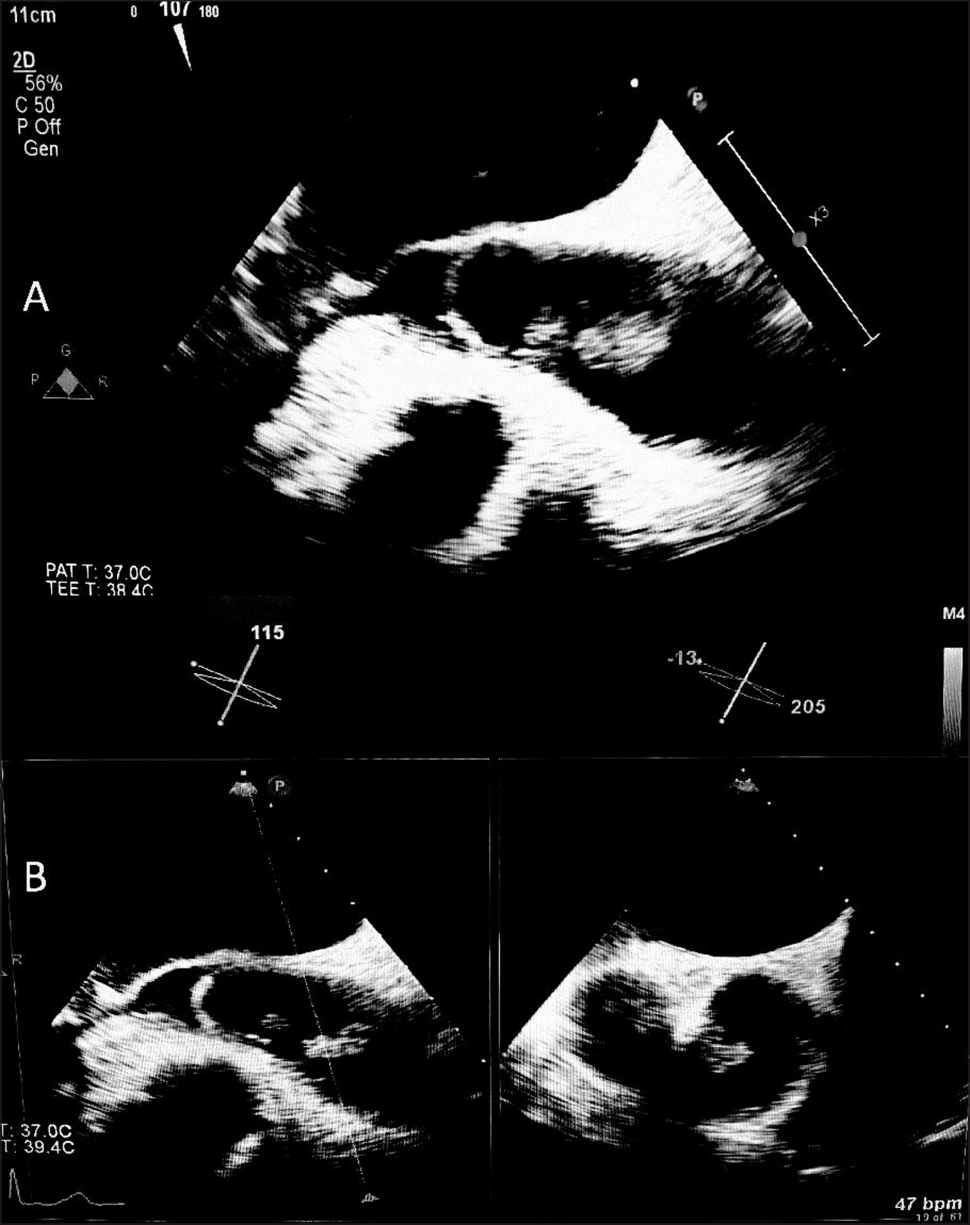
(A) Transesophageal view demonstrating a mobile pedunculated aortic mass in the ascending aorta, superior to the aortic valve. (B) The same aortic mass is visualized across an x-plane (perpendicular plane) view of the transesophageal echocardiogram.
